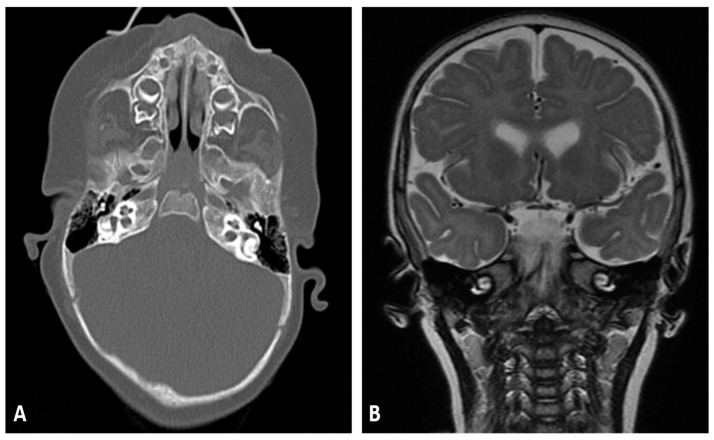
High-resolution temporal bone imaging. (A) Axial CT scan showing normal cochlear, vestibular, and middle-ear anatomy without bony abnormalities or malformations. (B) MRI of the internal auditory canal demonstrating normal cochlear nerve morphology and intact vestibulocochlear structures, with no evidence of nerve deficiency or other structural anomalies.
